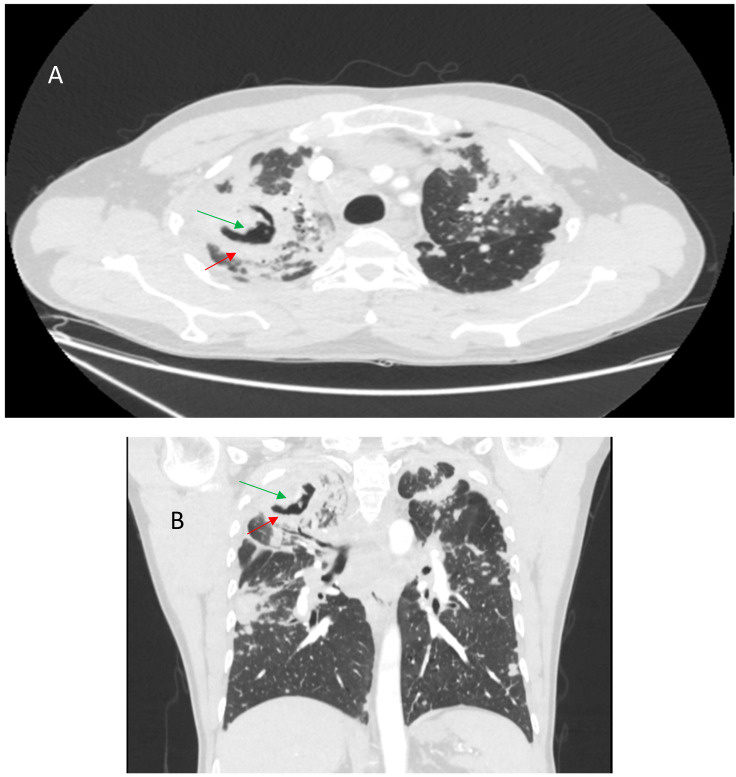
Axial CT scan (A) and coronal reconstruction (B) showing thick-walled cavity (red arrows) with mycetoma (green arrows) in the right upper lung lobe.
